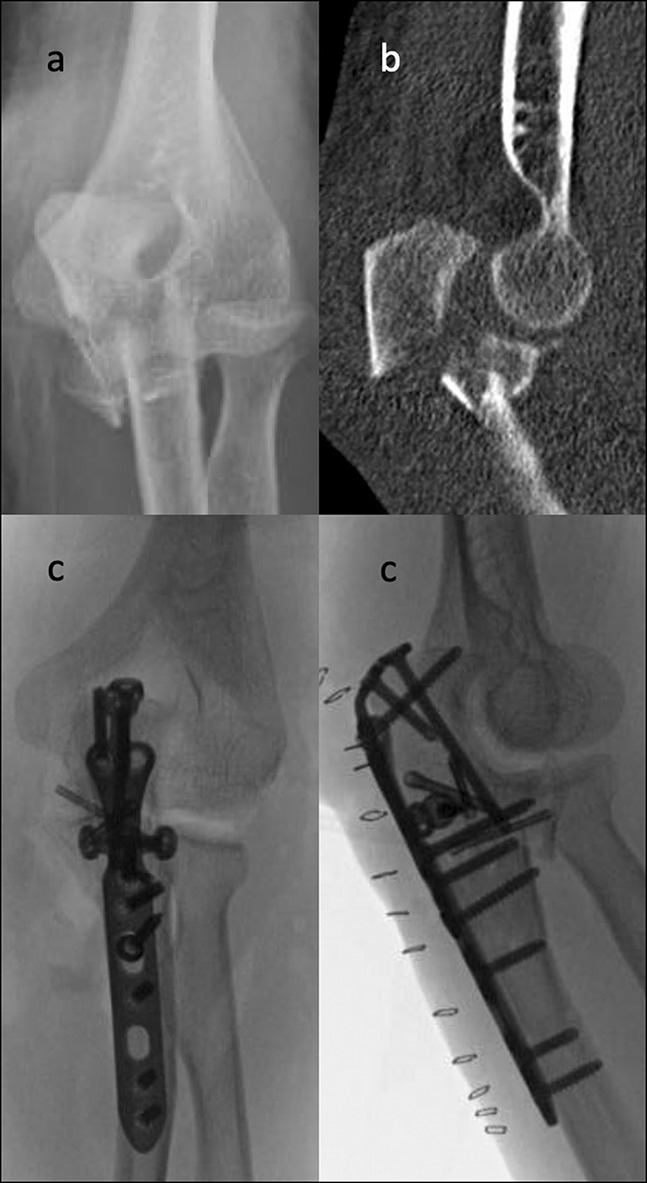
Radiology images (A) and CT scan (B) demonstrating transolecranon fracture-dislocation of the elbow. Radiographs demonstrating surgical fixation using a proximal ulna plate and screws (C).
